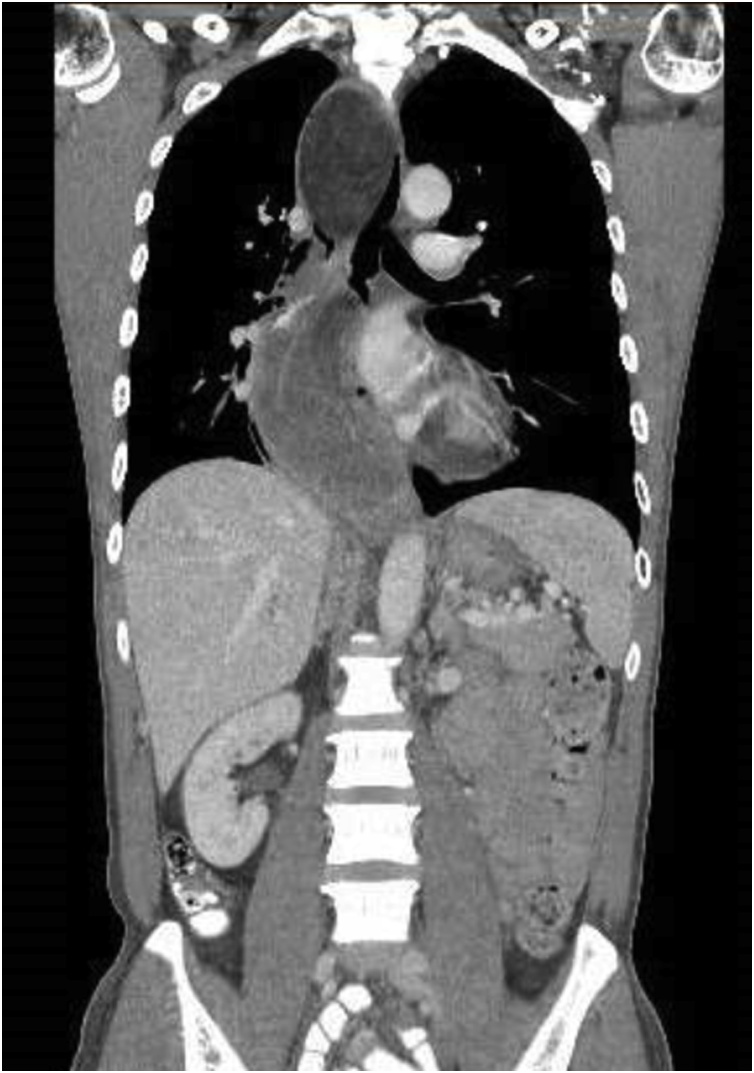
CT showing the heterogenous polypoidal mass.
